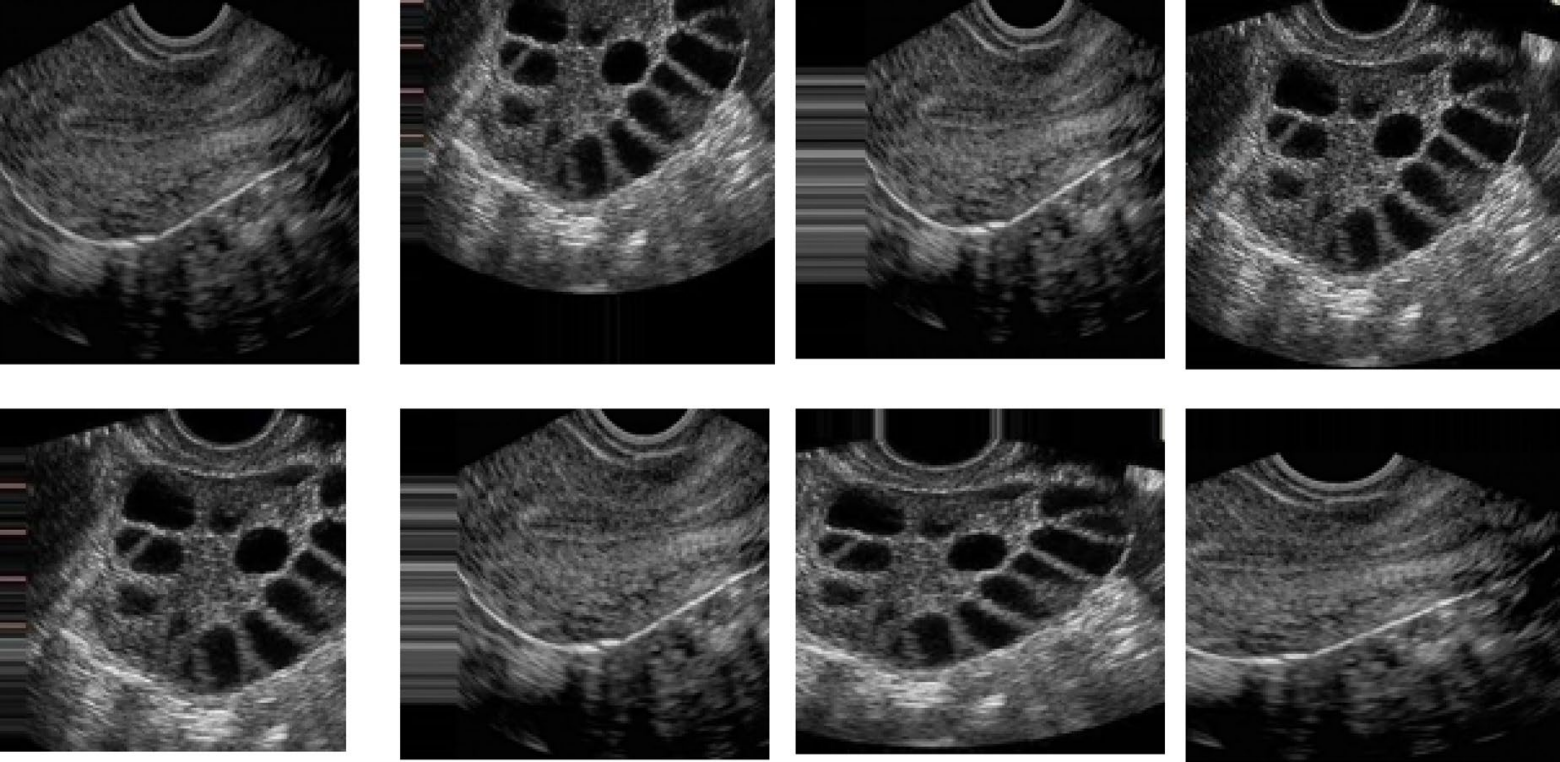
Sample PCOS Ultrasound Dataset Images.
